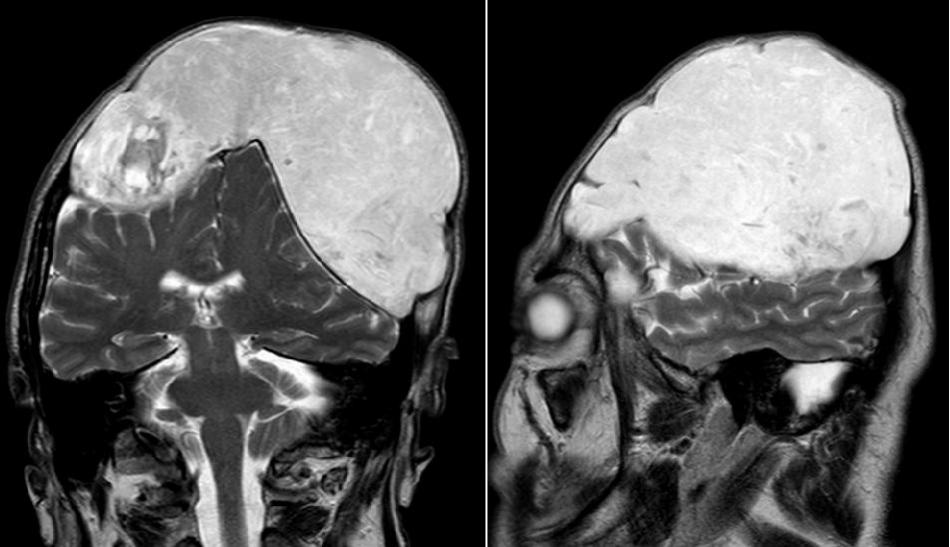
Magnetic resonance imaging T2 weighted images show the distinct compression of both brain hemispheres and ventricles due to the tumor, measuring 15 × 12 × 10 cm. An inhomogeneous high signal is shown within the tumor.
